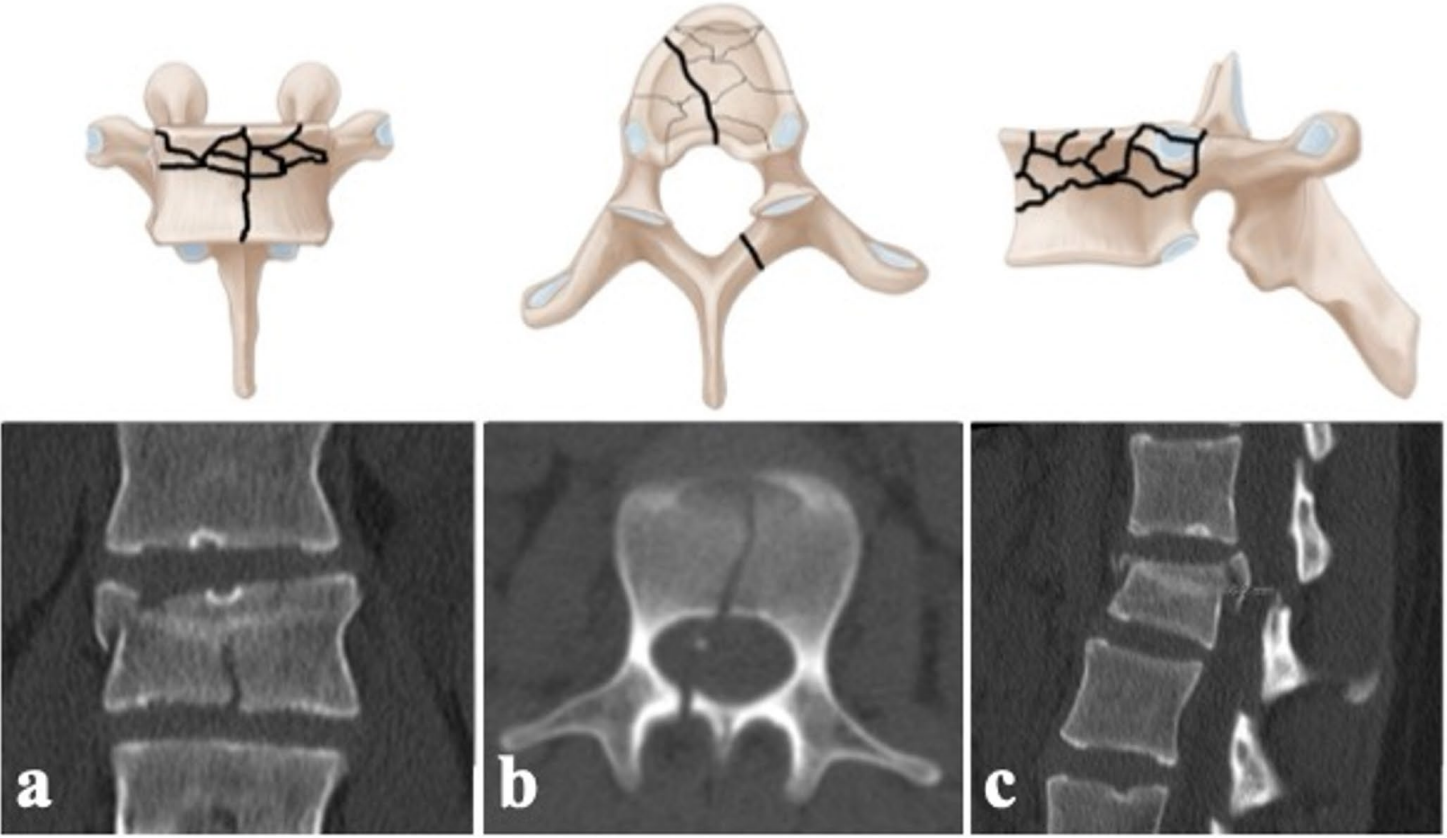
Schemes and CT-scans representing a burst-split fracture (AO Spine A4 type) in (a) anteroposterior, (b) axial, and (c) sagittal views
